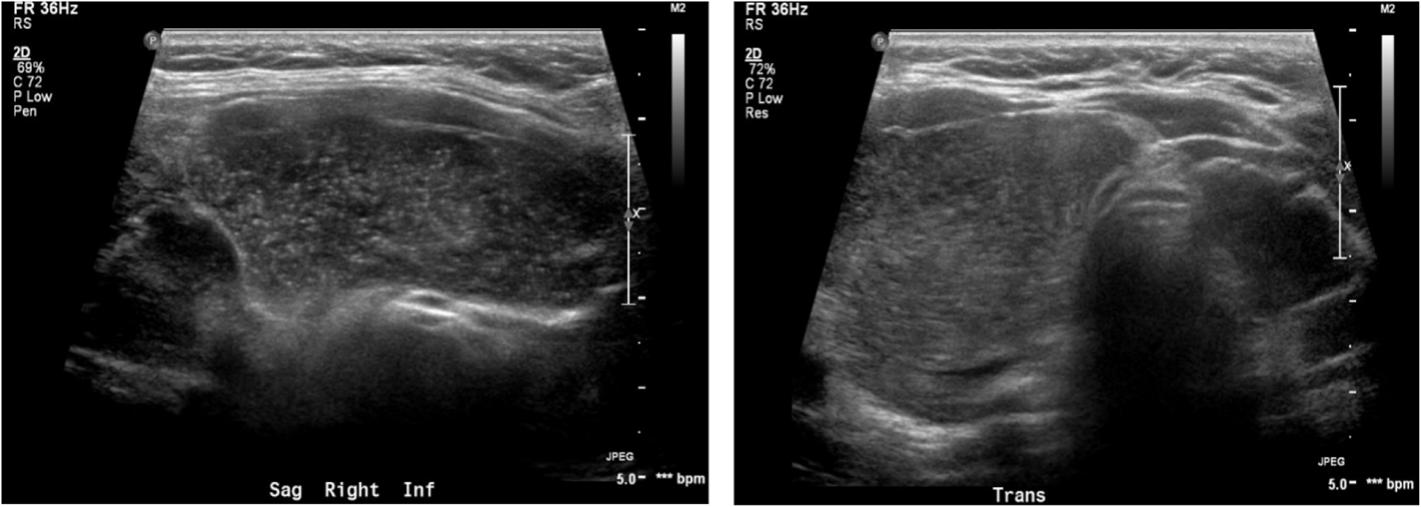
Ultrasound of the neck. Showing a large complex cystic lesion in the mid to lower portion of the right lobe. It measures about 4.9 cm × 2.3 cm × 4.8 cm
